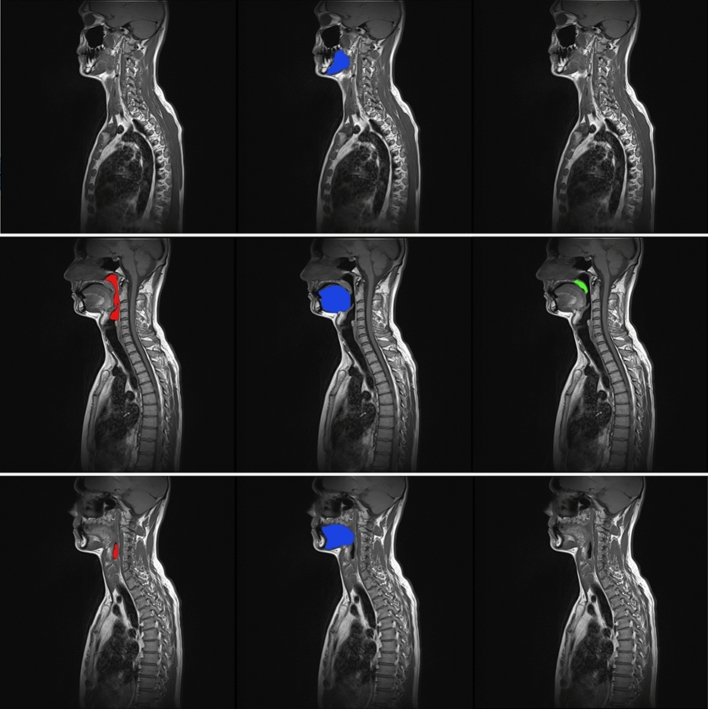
Example slices with overlaid manual segmentation of pharynx (left), tongue (middle), soft palate (right) with original T1-weighted slices 2, 7, 10 of one dataset
